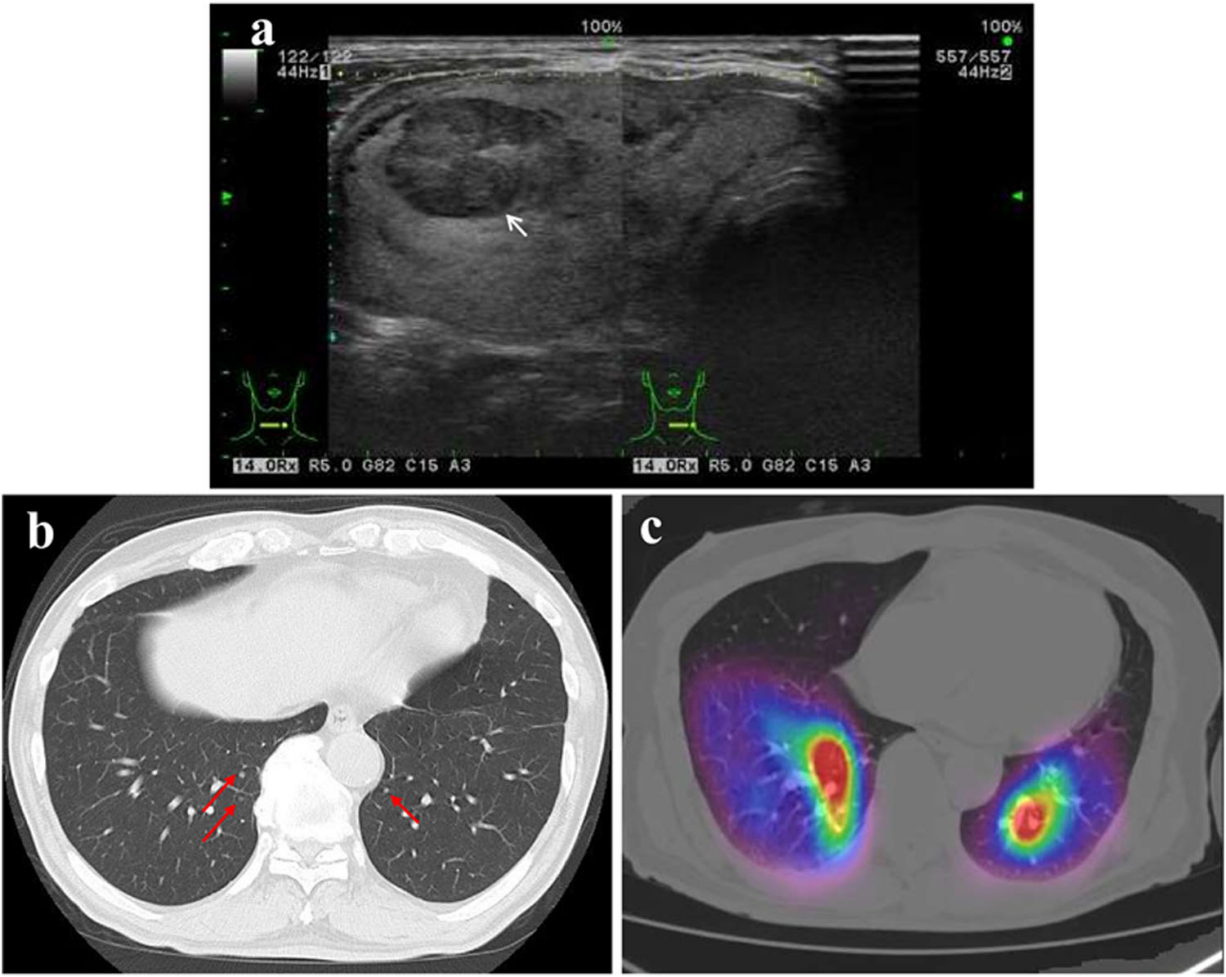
Clinical imaging findings. (a) Thyroid ultrasonography was performed before initial surgery, showing an isoechoic outer nodule and a hypoechoic inner nodule (white arrow). (b) Computed tomography performed after subcutaneous tumor enucleation suggests multiple small lung metastases. (c) 131‐I scan shows radioactive iodine uptake in the lungs.
